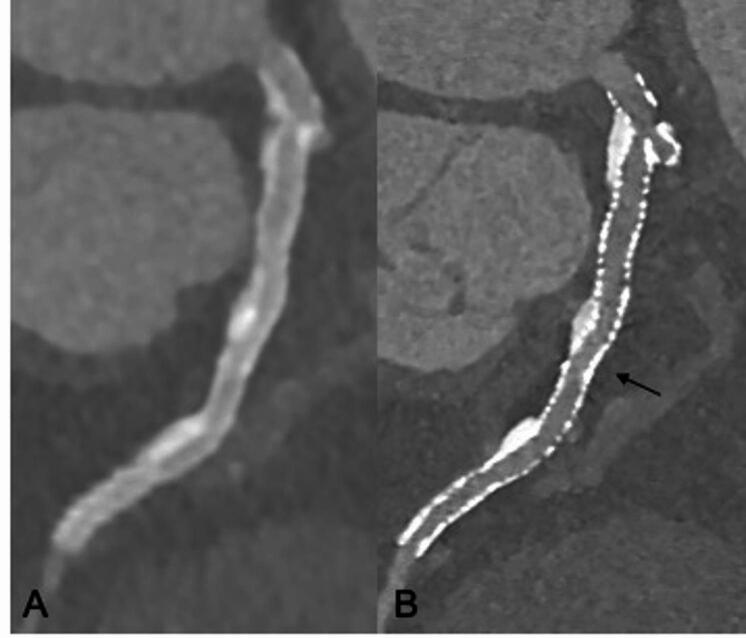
Stent Imaging on a novel Photon Counting Detector CT using (A) standard resolution scan mode and Bv 44 kernel vs. (B) Ultra High Resolution (UHR) scan mode and Bv 72 kernel. Due to the high spatial resolution and the sharp reconstruction kernels the stent structure is clearly visible – even in areas with multiple stent layers and the left main bifurcation – resulting in high diagnostic accuracy for stent assessment after a complex procedure.
